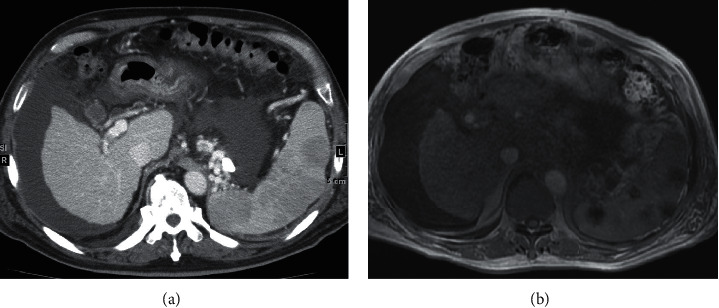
(a) CT with contrast revealed multiple small low-attenuation areas in the spleen. (b) MRI of the spleen revealed multiple low-intensity areas on T1-weighted imaging.
